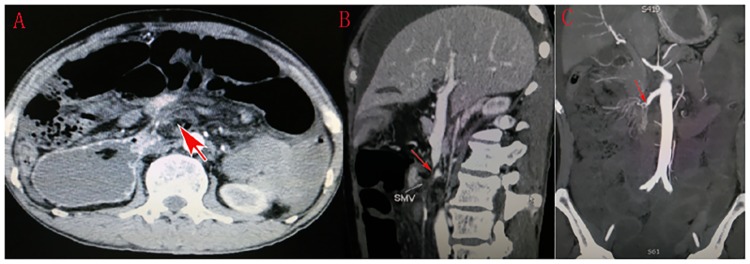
Three-dimensional abdominal CT showed the whirl sign of superior mesenteric vessels (A, red arrow). The longitudinal tapering of superior mesenteric vein (B) and artery (C) were found in the coronal scan.
